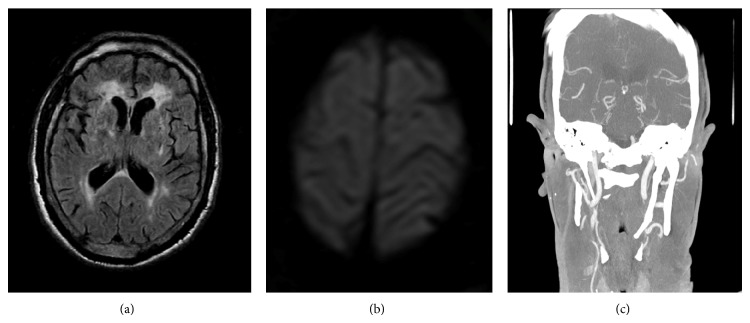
Pre-penicillin therapy imaging. (a) FLAIR, (b) DWI sequence, and (c) CT angiogram.
